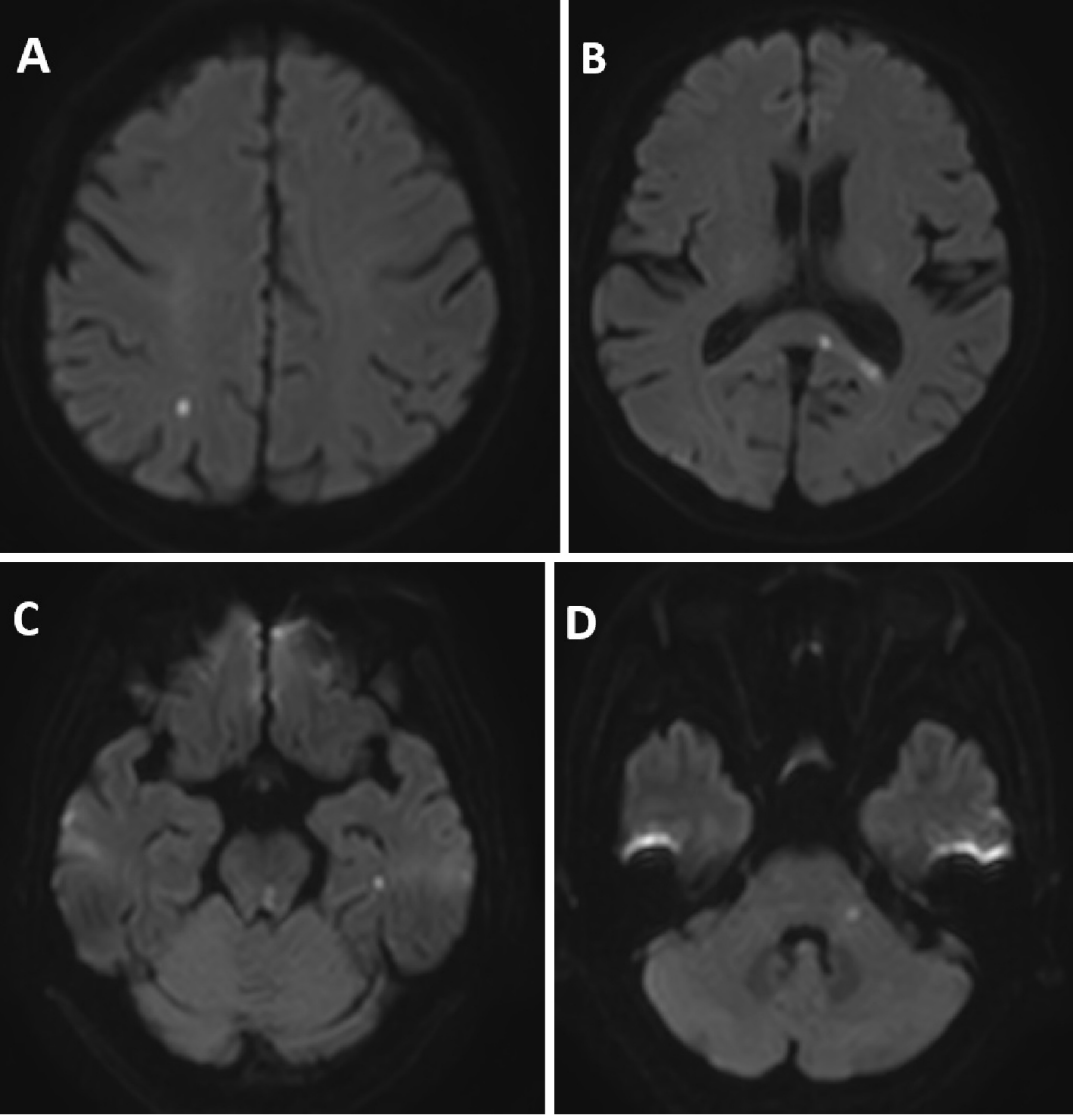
Diffusion-weighted magnetic resonance images obtained on admission showing multiple hyperintense lesions suggestive of acute infarctions. (A) Right parietal lobe. (B) Corpus callosum. (C) Left temporal lobe and dorsal part of the left pons, including the medial longitudinal fasciculus. (D) Left middle cerebellar peduncle.
